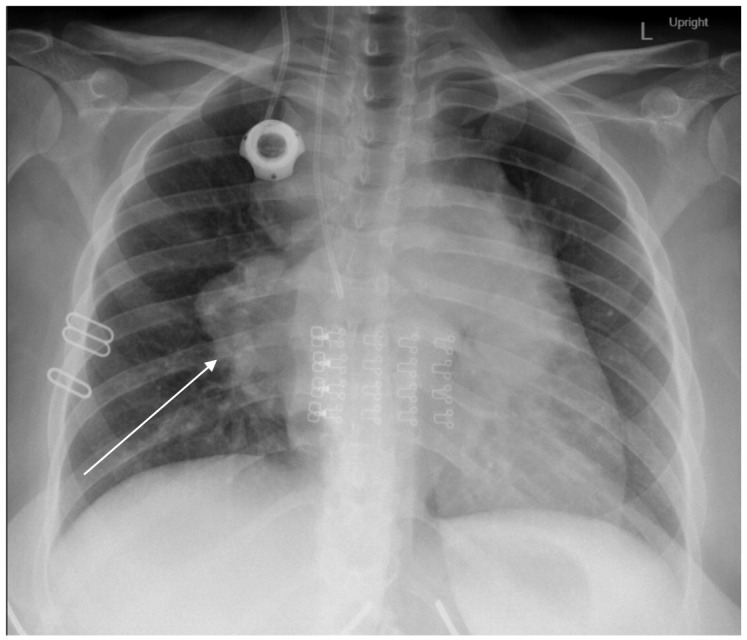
Patient’s chest x-ray at the time of diagnosis with an arrow showing bulky hilar adenopathy.
